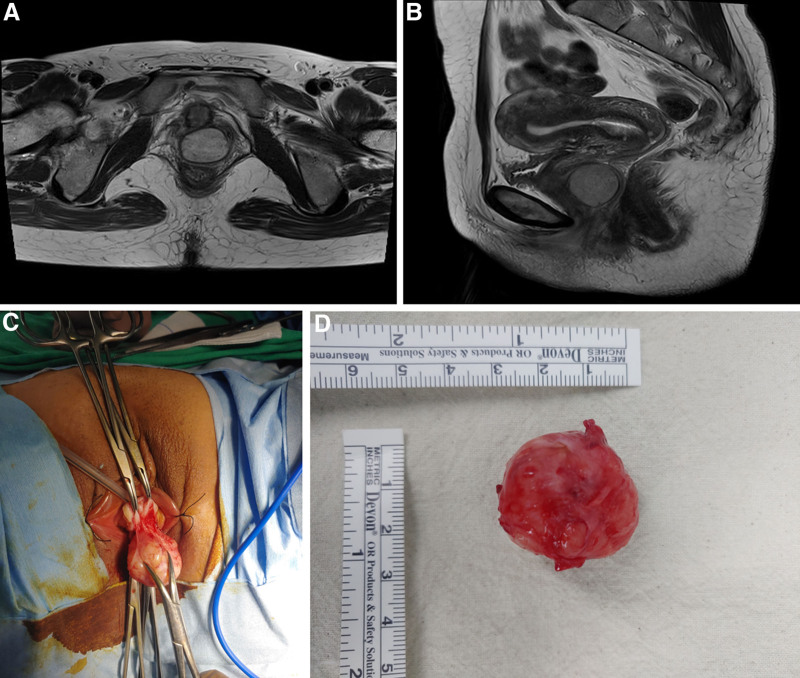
Pelvic magnetic resonance imaging (A) shows an approximately 2.5 cm round mass in the vagina on T2-weighted transverse image and (B) T2-weighted sagittal image. The mass was excised tranvaginally (C) and measured 3 × 3 cm in size grossly (D).
